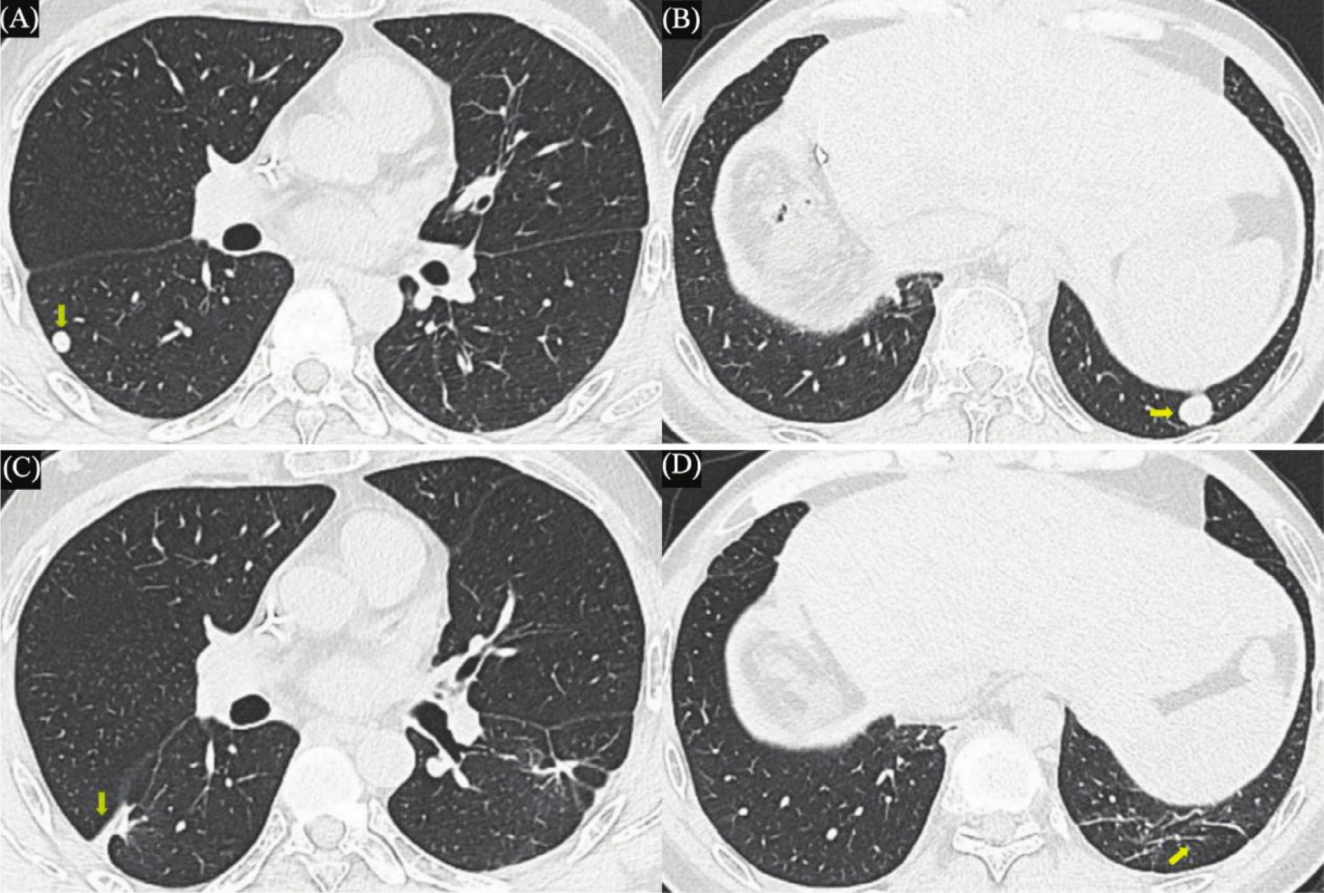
(A and B) CT scans reveal two de novo lung nodules (yellow arrow). (C and D) CT scans reveal the complete resection of two de novo lung nodules (yellow arrow).
